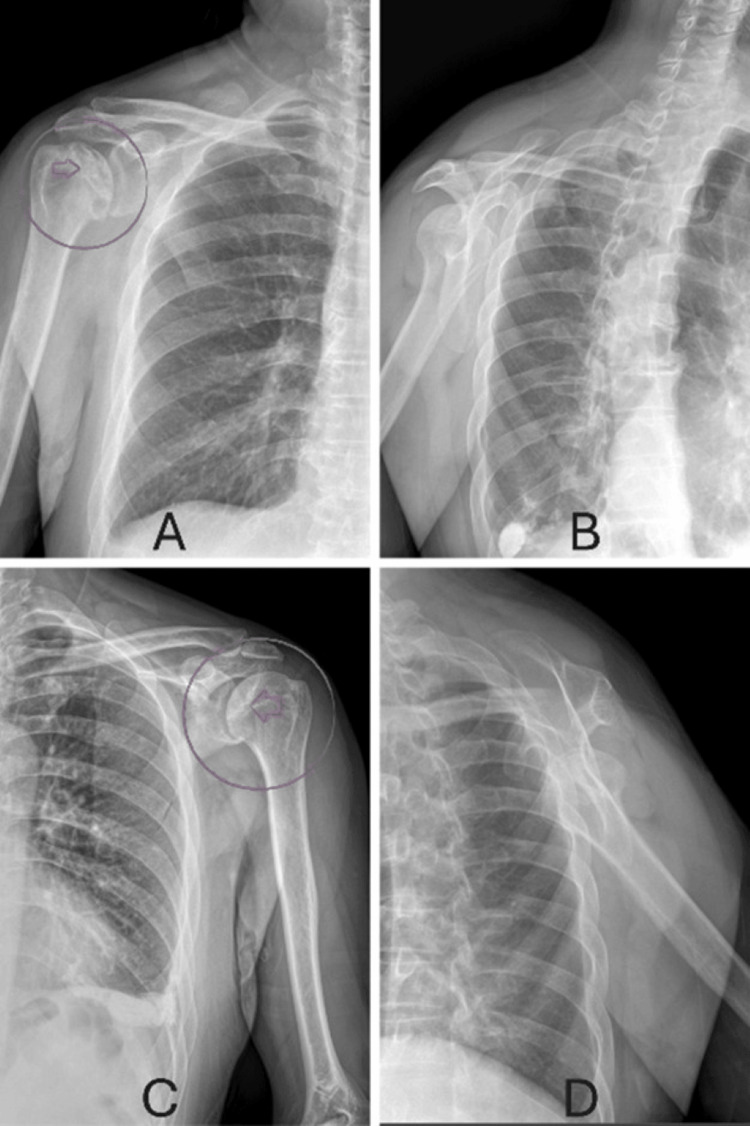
Radiographic findings of bilateral shoulder osteonecrosis (A) Anteroposterior radiograph of the right shoulder showing a crescentic subchondral radiolucent line (arrow, circled)
